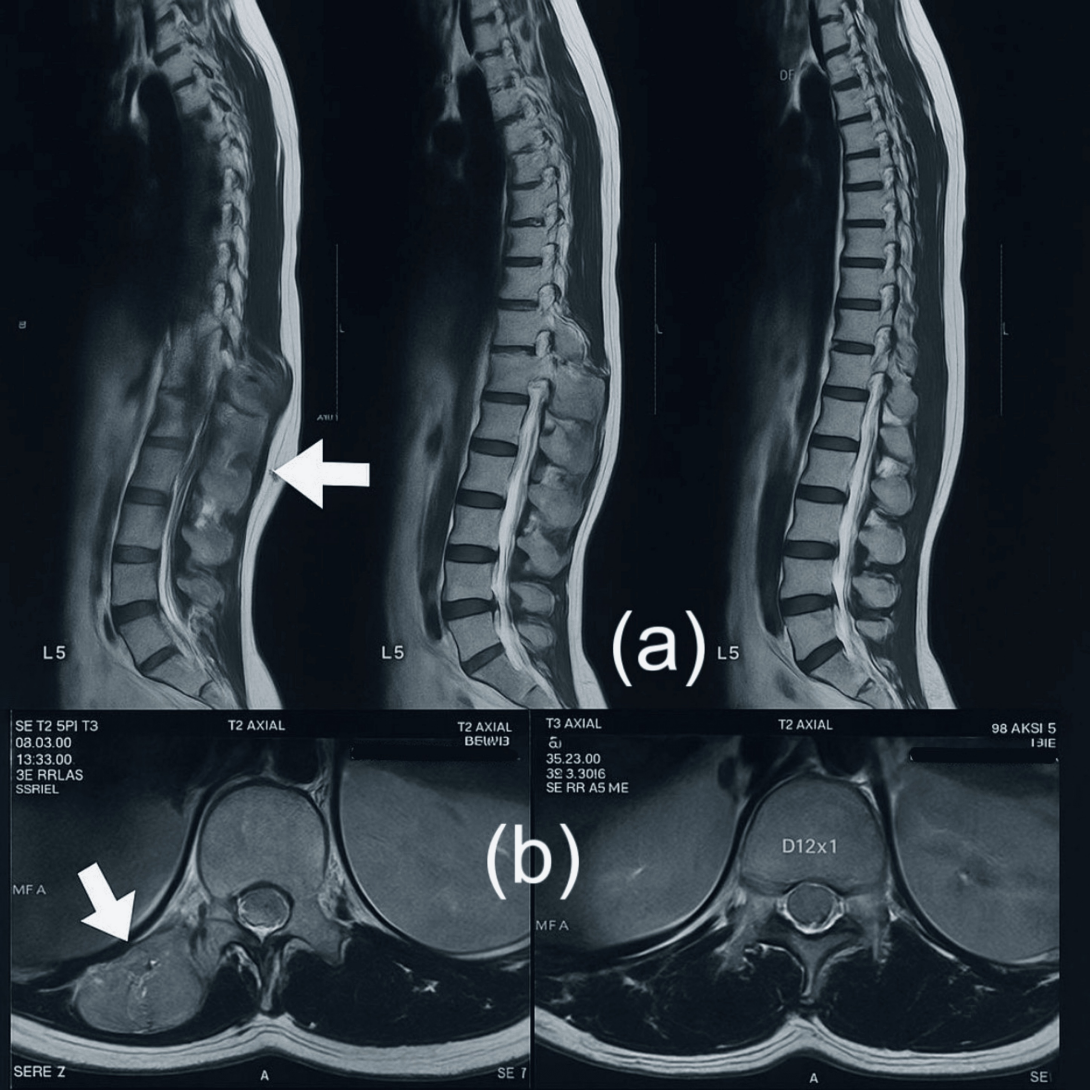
Sagittal and axial T2-weighted MRI images showing a heterogeneously enhancing extradural mass at T12 (white arrows), arising from the right neural foramen. The lesion extends into the right erector spinae muscle and causes bony erosion of the lamina, pedicle, and transverse process-mimicking a nerve sheath tumor (a) Sagittal T2-weighted MRI images of the thoracolumbar spine show a heterogeneously hyperintense extradural mass at the T12 level (white arrow), arising from the right neural foramen. (b) Axial T2-weighted MRI images demonstrate the lesion extending into the right erector spinae muscle (white arrow), with compression of the spinal canal and features resembling a nerve sheath tumor
